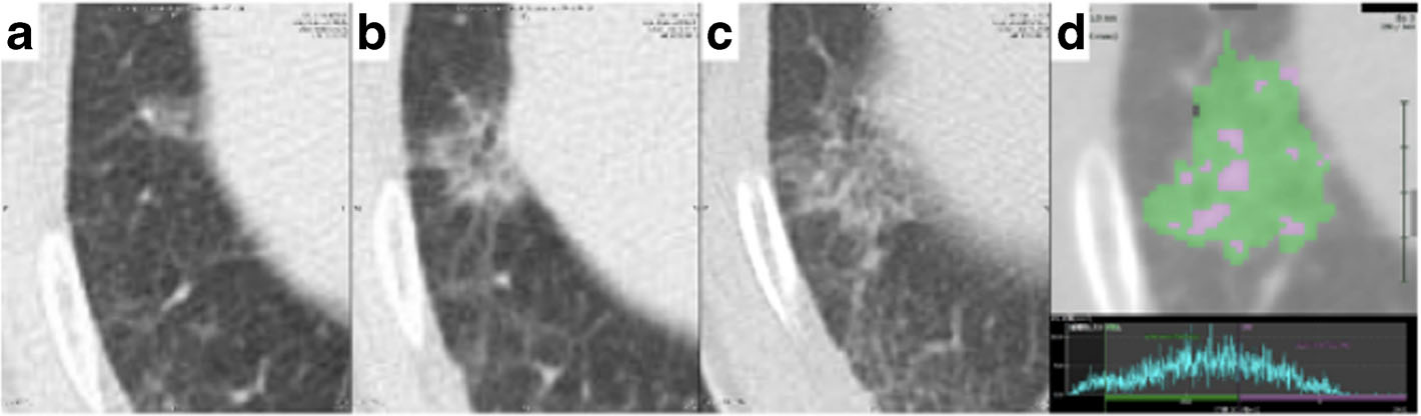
A series of computed tomography images of a squamous cell carcinoma with ground glass nodule. a Chest computed tomography (CT) image showing a 7-mm ground glass nodule (GGN) in the *right lower* lobe when the lesion was first noted. b Chest CT, obtained 1 year after a; the GGN has enlarged to 18 mm. c Chest CT image obtained 1.5 year after a; the GGN has enlarged to 24 mm and a solid component has developed. d In a semi-automated three-dimensional volumetric “GGN analysis” of c, the ground glass opacity component (*green area*, between −800 and −301 Hounsfield units) accounted for 64% of the tumor in contrast to the remaining solid component (*purple area*, ≧ −300 Hounsfield units)
